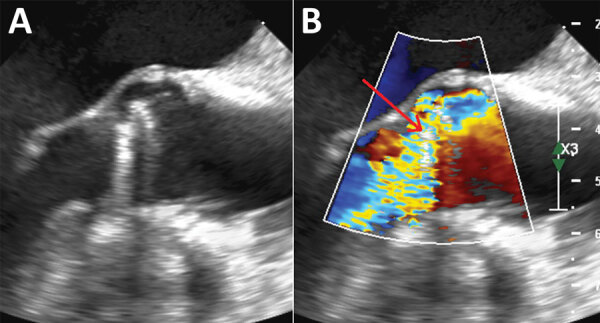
Trans-esophageal echocardiography without doppler (A) and with doppler (B) of the mechanical aortic heart valve in a woman diagnosed with *Mycoplasma genitalium* endocarditis. Red arrow indicates severe paraprosthetic regurgitation.
